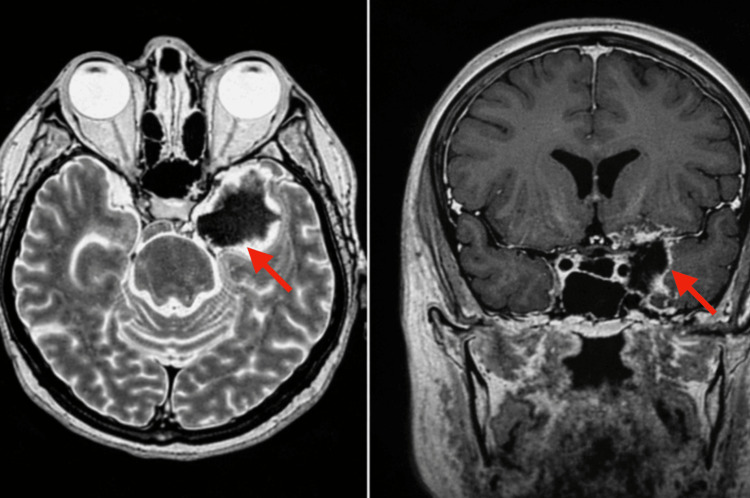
Postoperative Follow-up MRI Demonstrating Debulking of a Left Cavernous Sinus Lesion Axial T2-weighted MRI (left) and coronal T1-weighted post-contrast MRI (right) obtained during postoperative follow-up demonstrate interval debulking of the previously identified lesion in the left cavernous sinus. Imaging shows postoperative changes with reduction of the mass effect and no radiographic evidence of residual tumor (red arrows).
